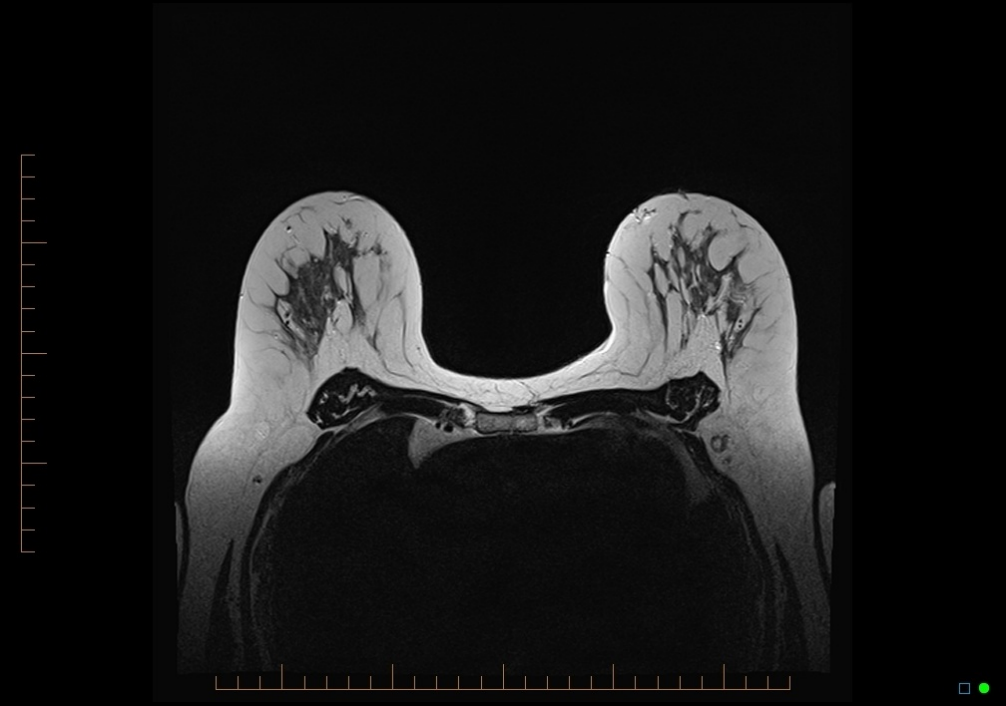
MRI breast: T2 axial, no suspicious axillary lymph nodes MRI: magnetic resonance imaging
